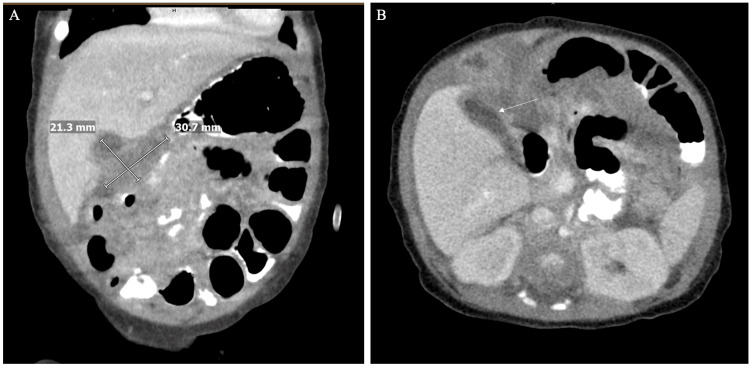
Computed tomography of the abdomen and pelvis with IV and oral contrast. (A) Coronal view and (B) axial view confirming liver abscesses with surrounding fluid collections and inflammatory changes.
